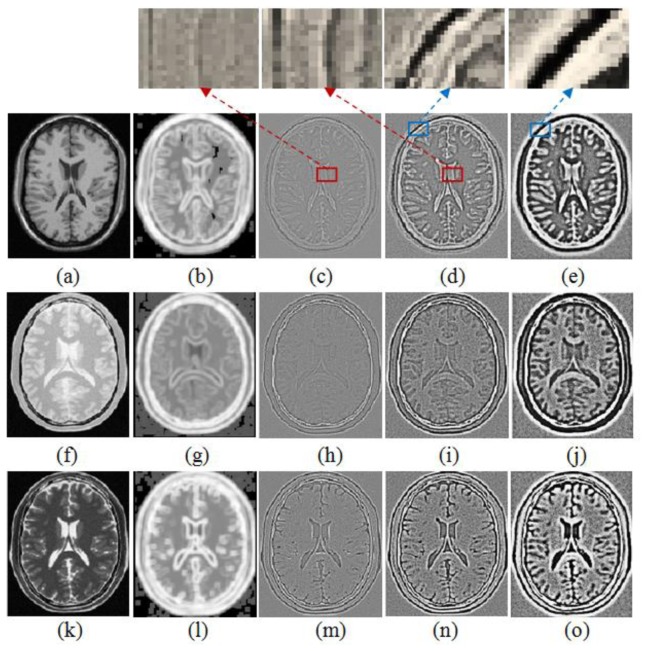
Entropy image and WLD with different *R* of the various MR images. (a) deformed T1 image; (b) Entropy image of (a); (c) WLD with *R* = 1 of (a); (d) WLD with *R* = 2 of (a); (e) WLD with *R* = 3 of (a); (f) deformed PD image; (g) Entropy image of (f); (h) WLD with *R* = 1 of (f); (i) WLD with *R* = 2 of (f); (j) WLD with *R* = 3 of (f); (k) original T2 image; (l) Entropy image of (k); (m) WLD with *R* = 1 of (k); (n) WLD with *R* = 2 of (k); (o) WLD with *R* = 3 of (k).
